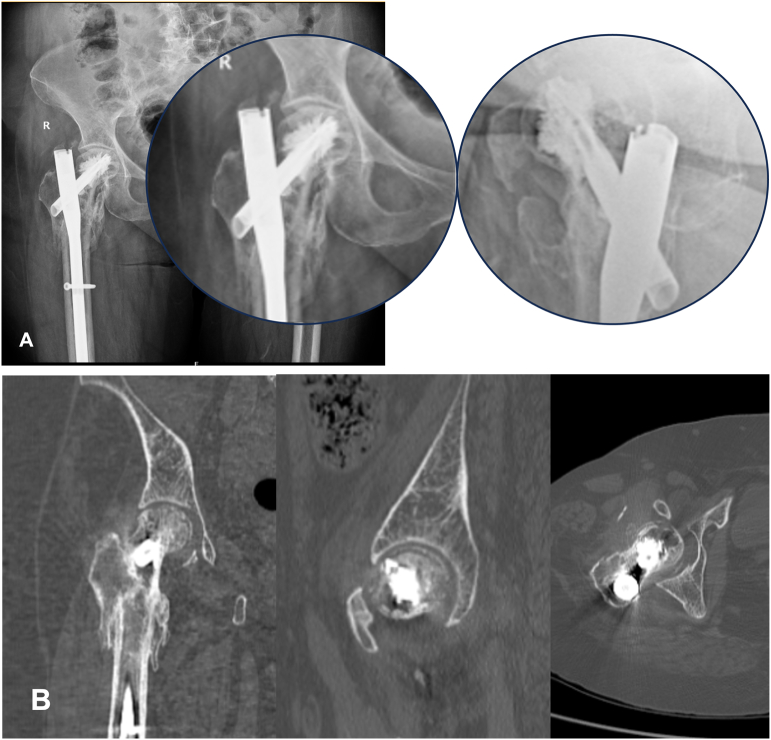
A) 18th month Post op x ray AP and lateral View showing Changes of ONFH– subchondral bone collapse and subchondral cysts. b): CT scan hip showing Changes of ONFH with crescent sign.
